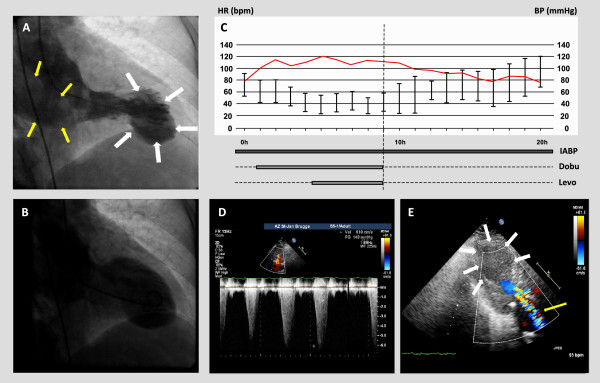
Diagnostic evaluation and treatment of a patient with takotsubo cardiomyopathy and severe left ventricular outflow tract (LVOT) obstruction. A 74-year old woman was admitted with ST-segment elevation in the precordial leads and in cardiogenic shock. Panel A-B: Left ventricular angiography shows a typical pattern of ‘apical ballooning’ at systole (panel A) when compared to diastole (panel B). Panel C: Intra-aortic balloon pump counter-pulsation therapy was initiated in the cath-lab. Because of refractory shock, dobutamine (dobu) and norepinephrine (levo) were started at the ICU. Cessation of inotropic therapy after echocardiographic diagnosis of LVOT obstruction resulted in recovery of blood pressure. Panel D: Severe LVOT obstruction was identified on continuous wave Doppler echocardiography (end-systolic pressure gradient 149 mmHg). Panel E: Echocardiography confirming the presence of apical akinesia or ‘apical ballooning’. HR: heart rate (beats per minute); BP: blood pressure (mmHg). The white arrows indicate ‘apical ballooning’; the yellow arrows indicate systolic anterior motion (SAM)-induced mitral regurgitation.
